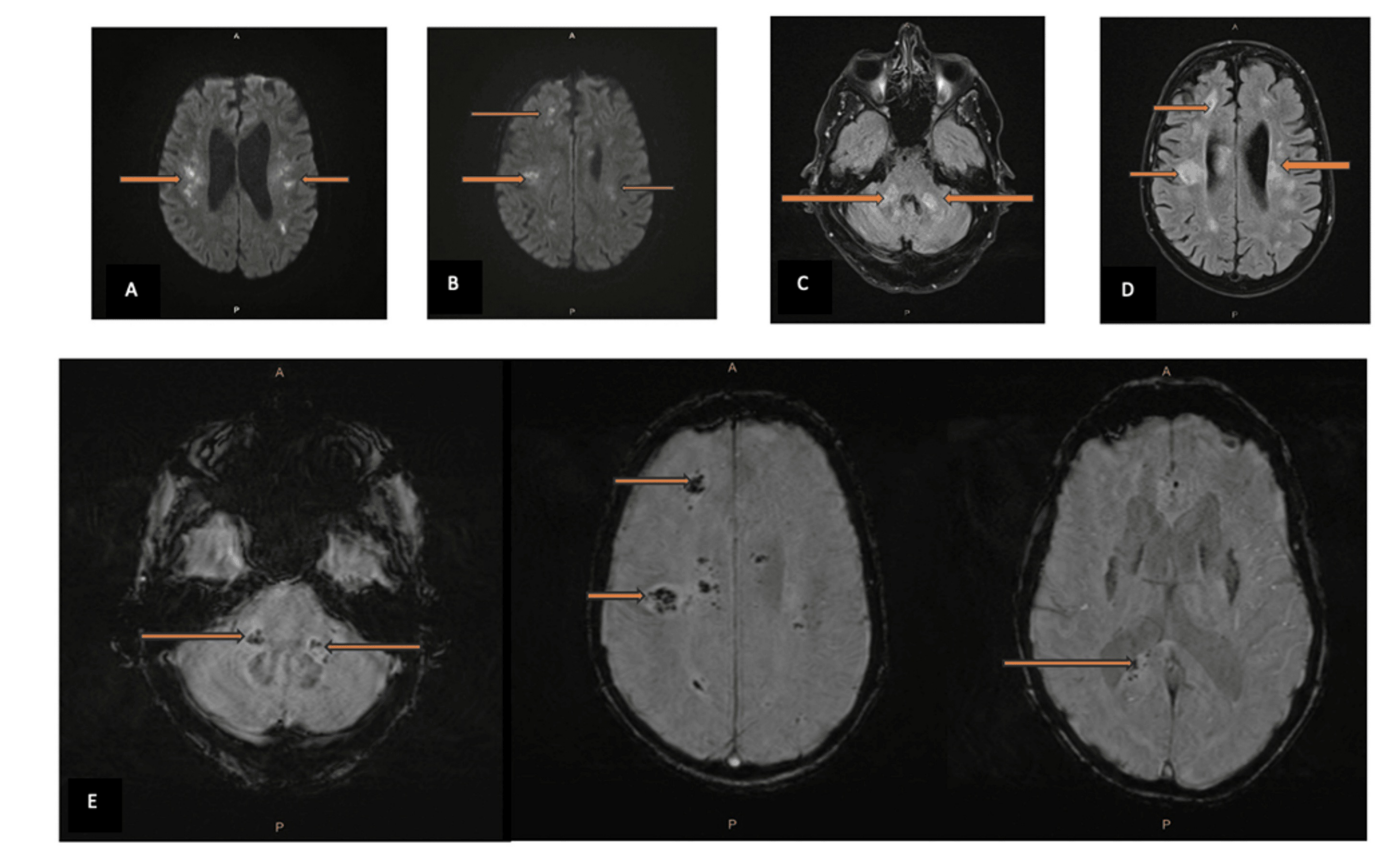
(A, B) Brain MRI diffusion-weighted images (DWIs): high SI denoting true restricted diffusion (arrows). (C, D) Brain MRI T2 FLAIR: showing high T2 and FLAIR signal distribution in cerebral hemispheres and white matter of the posterior fossa in the cerebral peduncles (arrows). (E) Brain MRI susceptibility-weighted images (SWIs): showing microhemorrhages (arrows). SI: signal intensity, FLAIR: fluid-attenuated inversion recovery.
